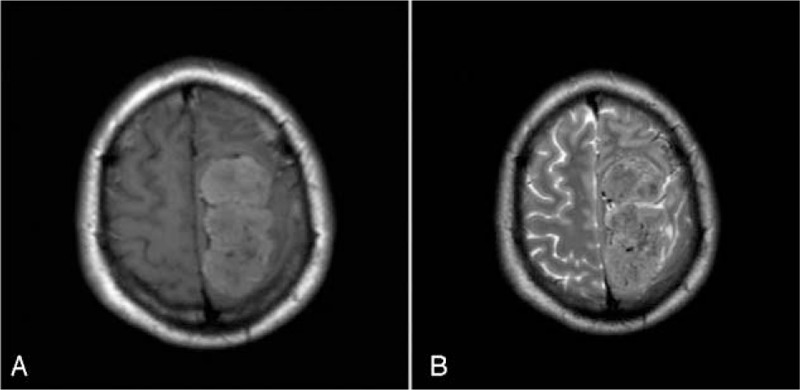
Brain MRI revealed (A) high mixed-intensity signals on the T1-weighted MRI, and (B) high mixed-intensity signals on the T2-weighted MRI. MRI = magnetic resonance imaging.
